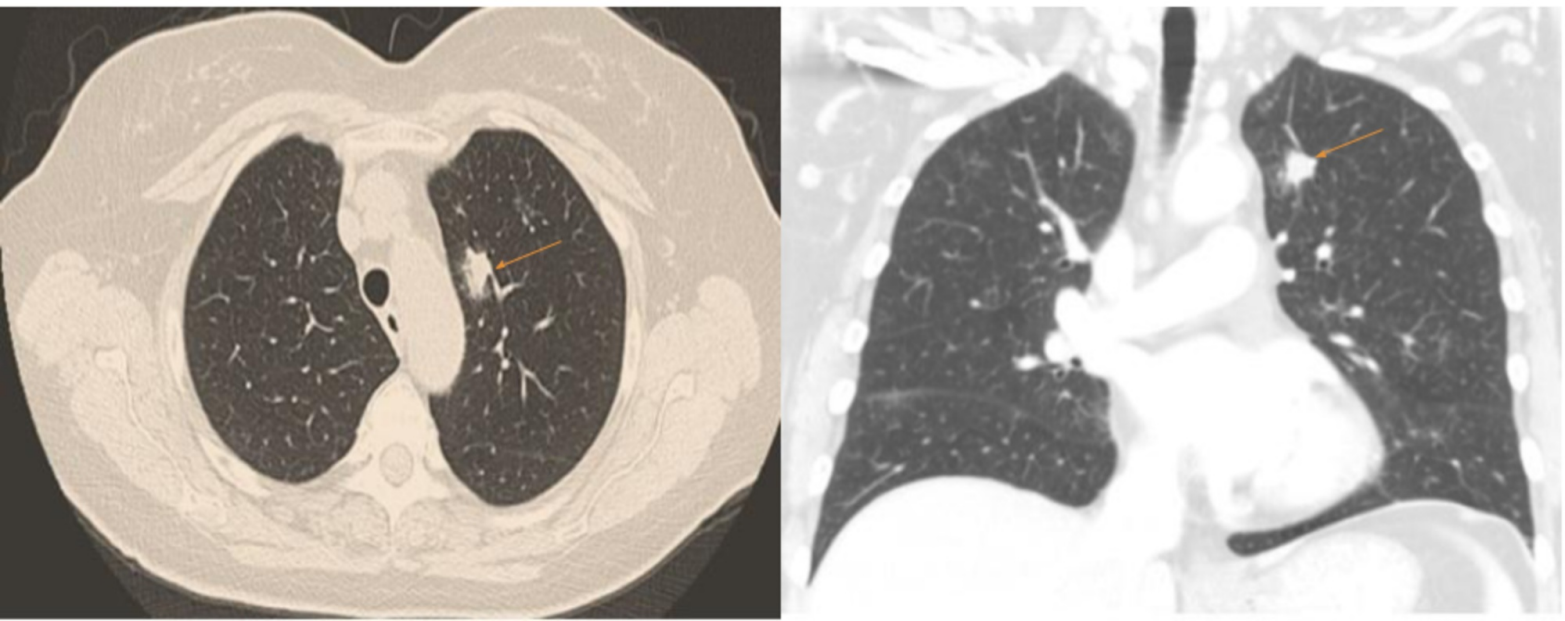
Computed tomography (CT) of the chest showing 2.1 x 0.9 cm left upper lobe nodule
